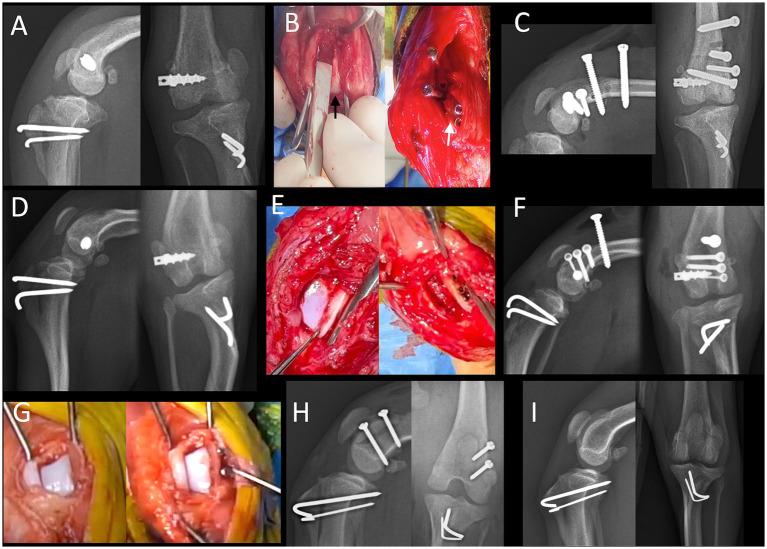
Intraoperative photographs and pre- and postoperative radiographs. (A,C) Mediolateral and craniocaudal radiographs from Case 1. (A) Preoperative views showing medial patellar re-luxation; no definitive fracture line of the trochlear ridge was clearly identified on standard radiographs. (C) Immediate postoperative views demonstrating placement of three subfragmentary buttress screws beneath the trochlear ridge fragment and two additional screws placed to counteract medialising forces. (B) Intraoperative photographs from Case 1. Left: fracture line of the medial trochlear ridge prior to fixation (black arrow). Right: reduction of the fragment (white arrow) and subsequent screw fixation. (D,F) Mediolateral and craniocaudal radiographs from Case 2. (D) Preoperative views demonstrating medial patellar re-luxation; no definitive fracture line of the trochlear ridge was clearly identified on standard radiographs. (F) Immediate postoperative views showing reduction of the medial trochlear ridge fragment and placement of subfragmentary buttress screws beneath the fragment and an additional screw placed to counteract medialising forces. (E) Intraoperative photographs from Case 2. Left: fractured medial trochlear ridge prior to fixation. Right: reduced and stabilized fragment after screw placement. (G) Intraoperative photographs from Case 3. Left: rotated lateral trochlear ridge fragment before reduction. Right: anatomically repositioned and stabilized fragment. (H) Immediate postoperative radiographs from Case 3. The screw head appears slightly prominent relative to the cortical surface. (I) Postoperative radiograph obtained 377 days after revision surgery (184 days after implant removal) in Case 3.
